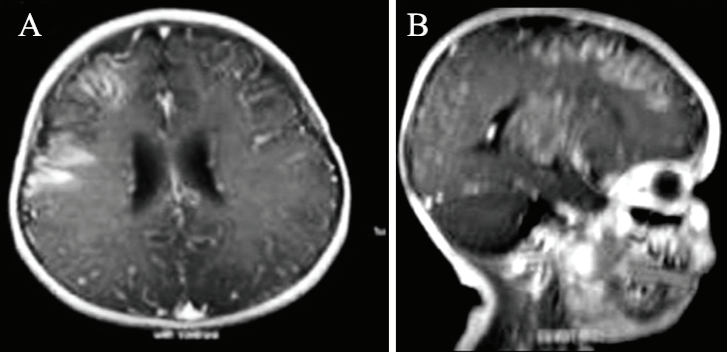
Axial (a) and coronal (b) post contrast T1-weighted images showing diffuse leptomeningeal enhancement (ivy sign) at the right frontoparietal region.
